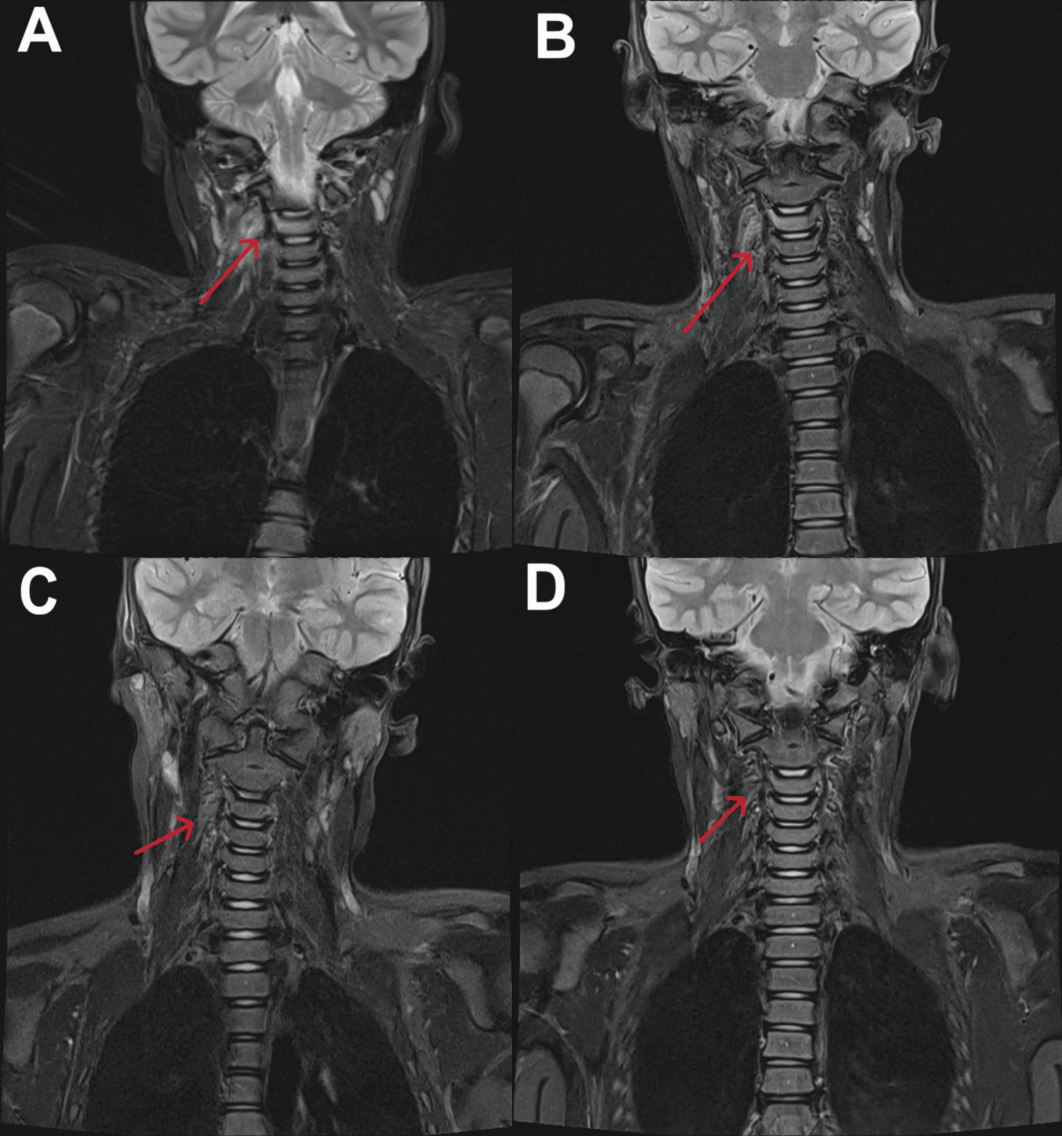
MRI images of the neck throughout the entire treatment course Image A - T2-weighted MRI of the neck showing hyperintensity involving the C3 vertebral body and right paravertebral soft tissues (arrow). No evidence of significant disc changes or adjacent collection was noted. Image B - T2-weighted MRI of the neck showing hyperintensity involving the C3 vertebral body, with mild, relatively decreased right-sided paravertebral inflammatory changes (arrow), without evidence of disease progression, necrotic changes, localised sizeable collection, or disc involvement. Image C - T2-weighted MRI of the neck showing a regressive course of the previously noted right paravertebral abnormal soft tissue signal (arrow). Image D - T2-weighted MRI of the neck showing no evidence of abnormal soft tissue signals or collections (arrow). No evidence of paravertebral soft tissue abnormal signal was noted. MRI, Magnetic Resonance Imaging
